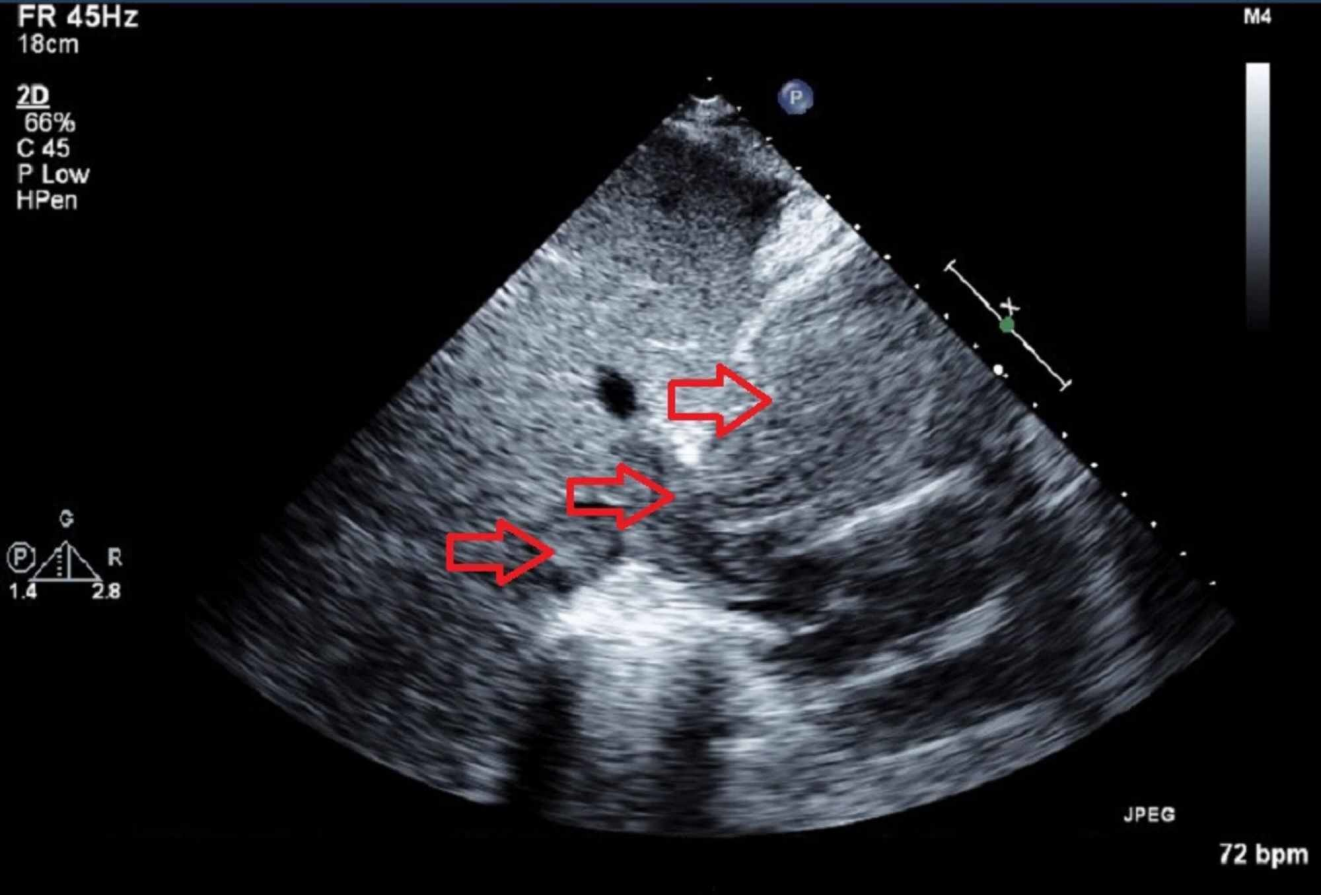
Subcostal transthoracic echocardiogram view shows the mass in the inferior vena cava and hepatic veins.
